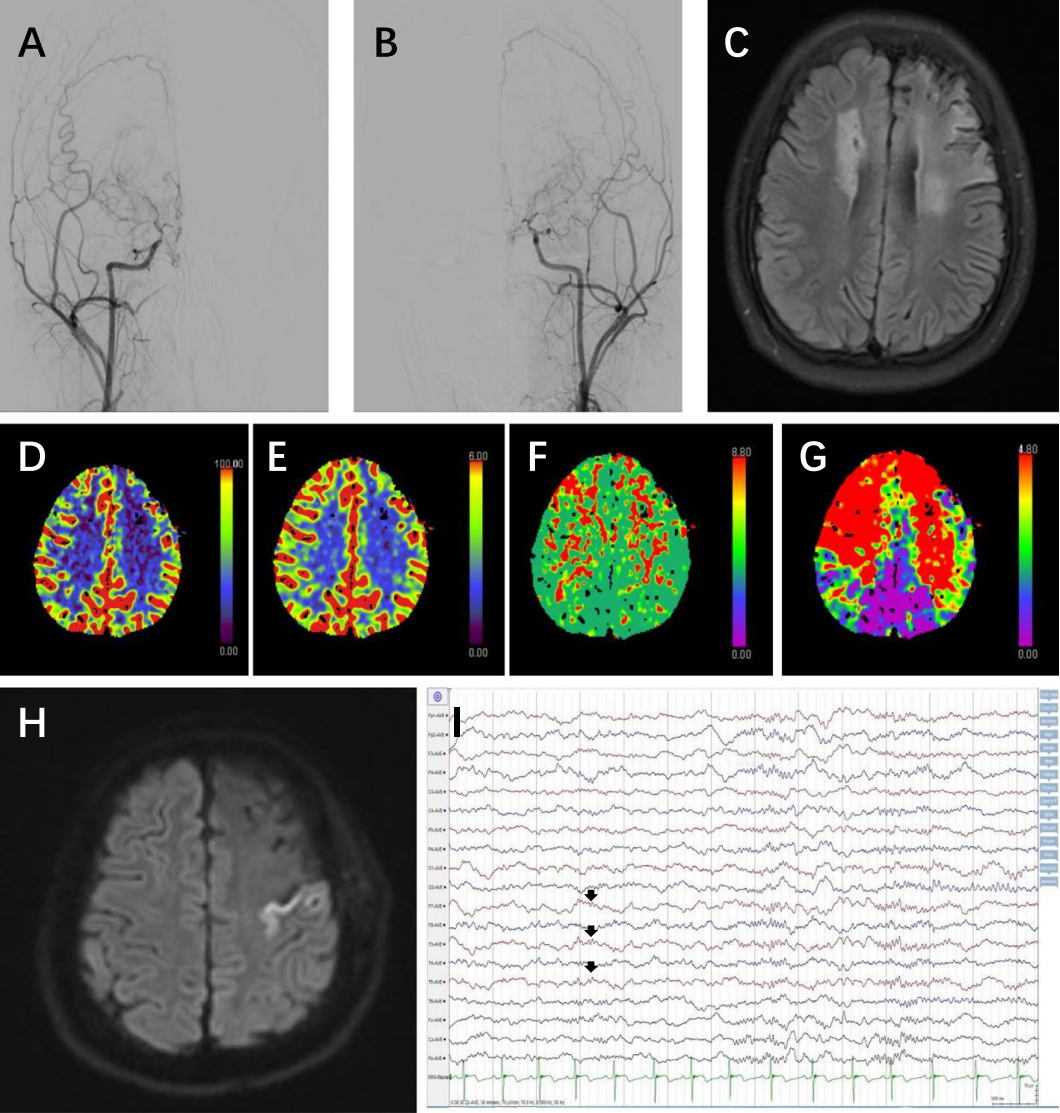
Case 9. Female, 14 years, left EDAS. One day after the operation, her right limb clonus and she was conscious. She was found to have cerebral infarction after the operation. A, B DSA showed occlusion of bilateral internal carotid arteries and formation of smoke-like vessels before operation. C Preoperative MRI revealed chronic cerebral infarction lesions in bilateral centrum semiovale and left frontal cortex. D–G Preoperative CTP (D CBF is roughly the same on both sides; E CBV is roughly the same on both sides; F MTT bilateral frontal lobe slows down; G Tmax bilateral frontal lobe slows down). H One day after operation, a high signal intensity of the left frontal lobe can be seen on the DWI sequence, and acute cerebral infarction is considered. I Postoperative VEEG showed that F7, T3, and T5 (left posterior temporal and frontal lobe) were visible Ɵ wave low amplitude slow wave activity (arrow)
